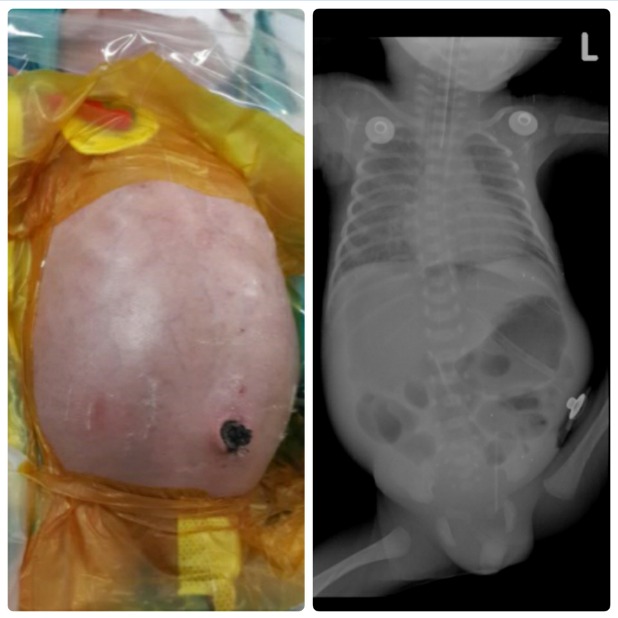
Figure 1: The abdominal characteristics and the radiograph of the baby at six days of age with distension of the abdomen and pneumoperitoneum.
